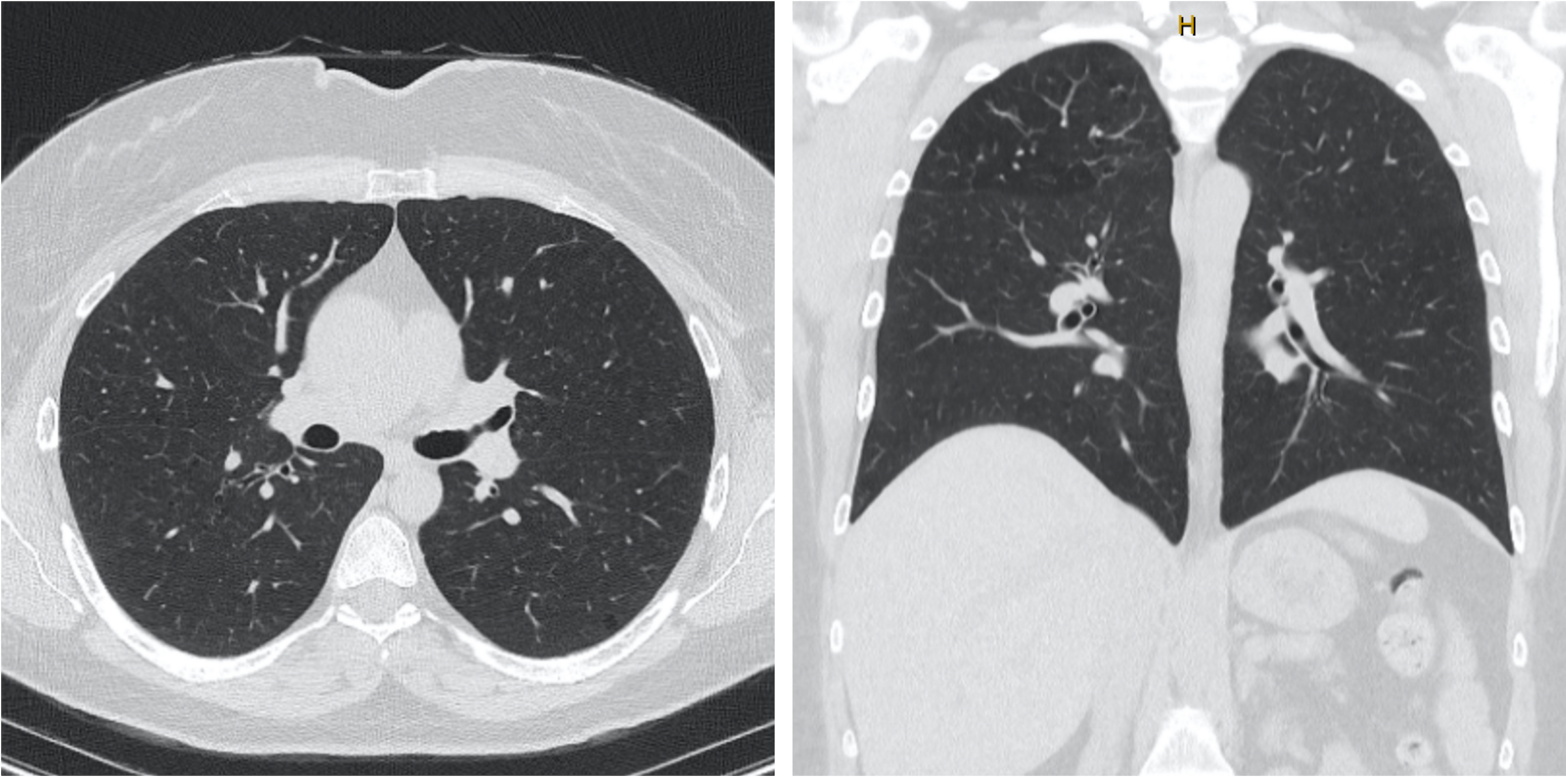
Transverse and coronal slices of repeat computed tomography chest 6 months after diagnosis, showing improvement in the number of pulmonary nodules.
